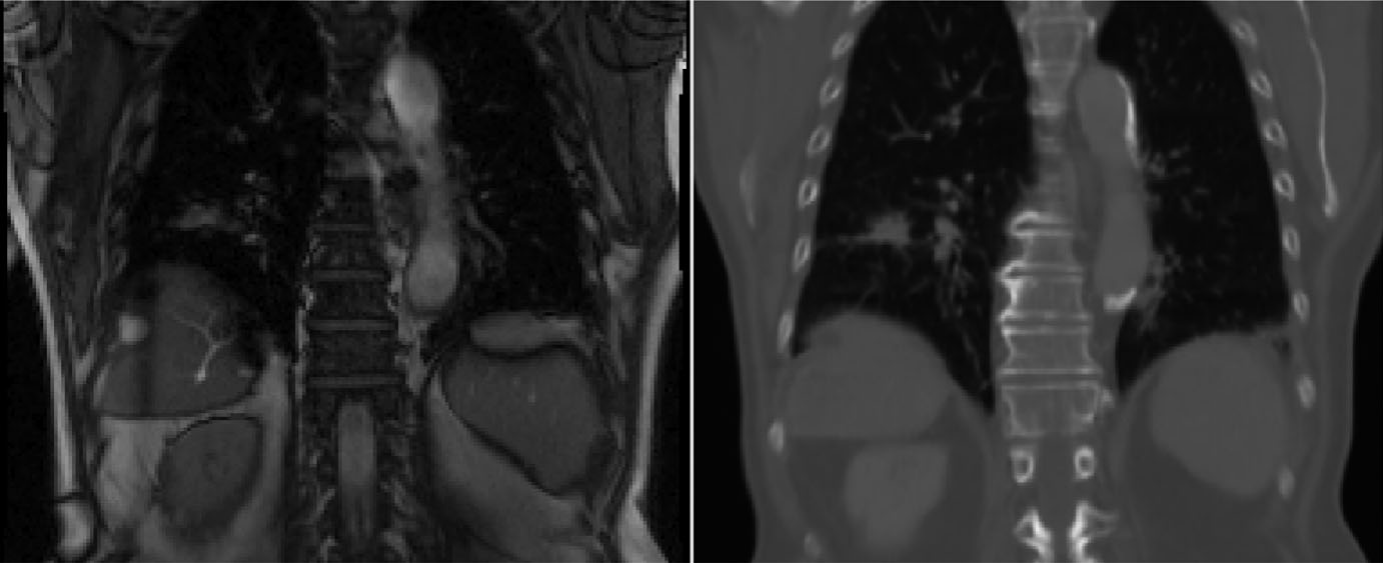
Example of 2DCT slice extraction after rigid registration of MRI on the 4DCTo.
